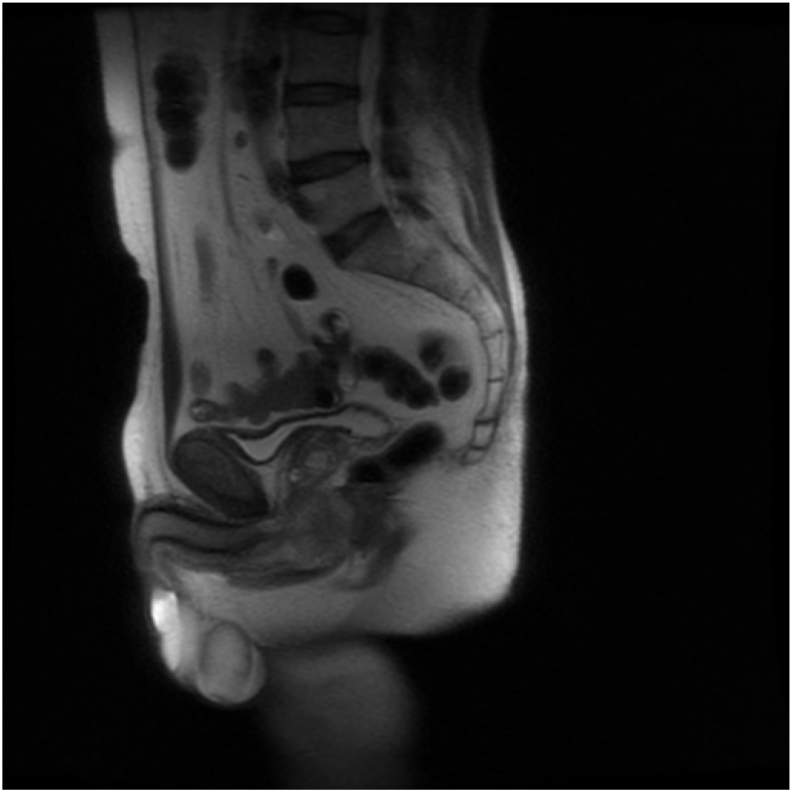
MRI Prostate, sagittal section.
